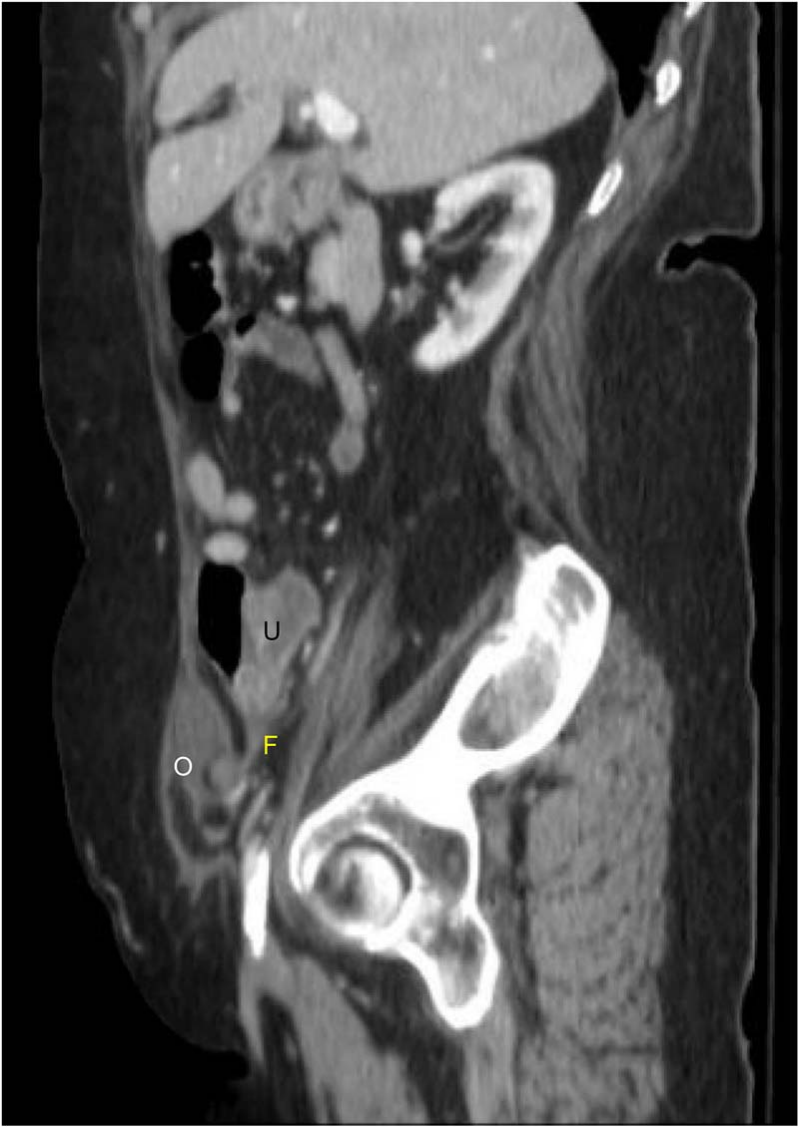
Sagittal view of contrast-enhanced computed tomography of the abdomen and pelvis demonstrating the right fallopian tube (F) attached to the uterus (U) extending into the Spigelian hernia defect containing the right ovary (O).
